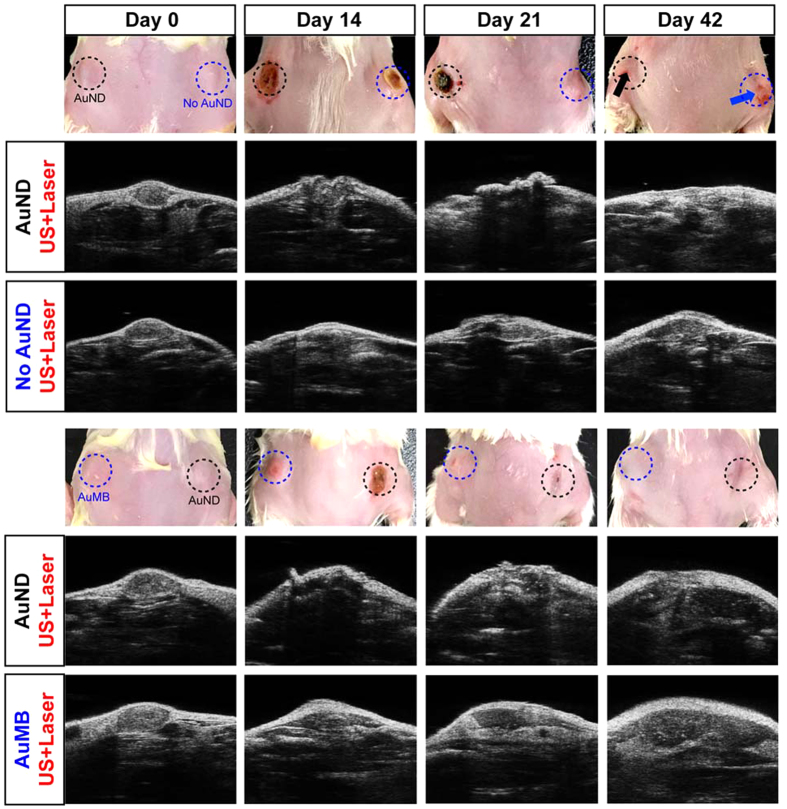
Enhanced therapeutic efficiency in tumors with AuNDs-assisted PPTT. Ultrasound B-mode imaging at the indicated treatment days shows that when tumors exposed to laser radiation and ultrasound simultaneously, the enhanced PPTT efficiency was observed in tumors loaded with AuNDs in compare to tumors loaded with AuMBs or without contrast agent loading.
